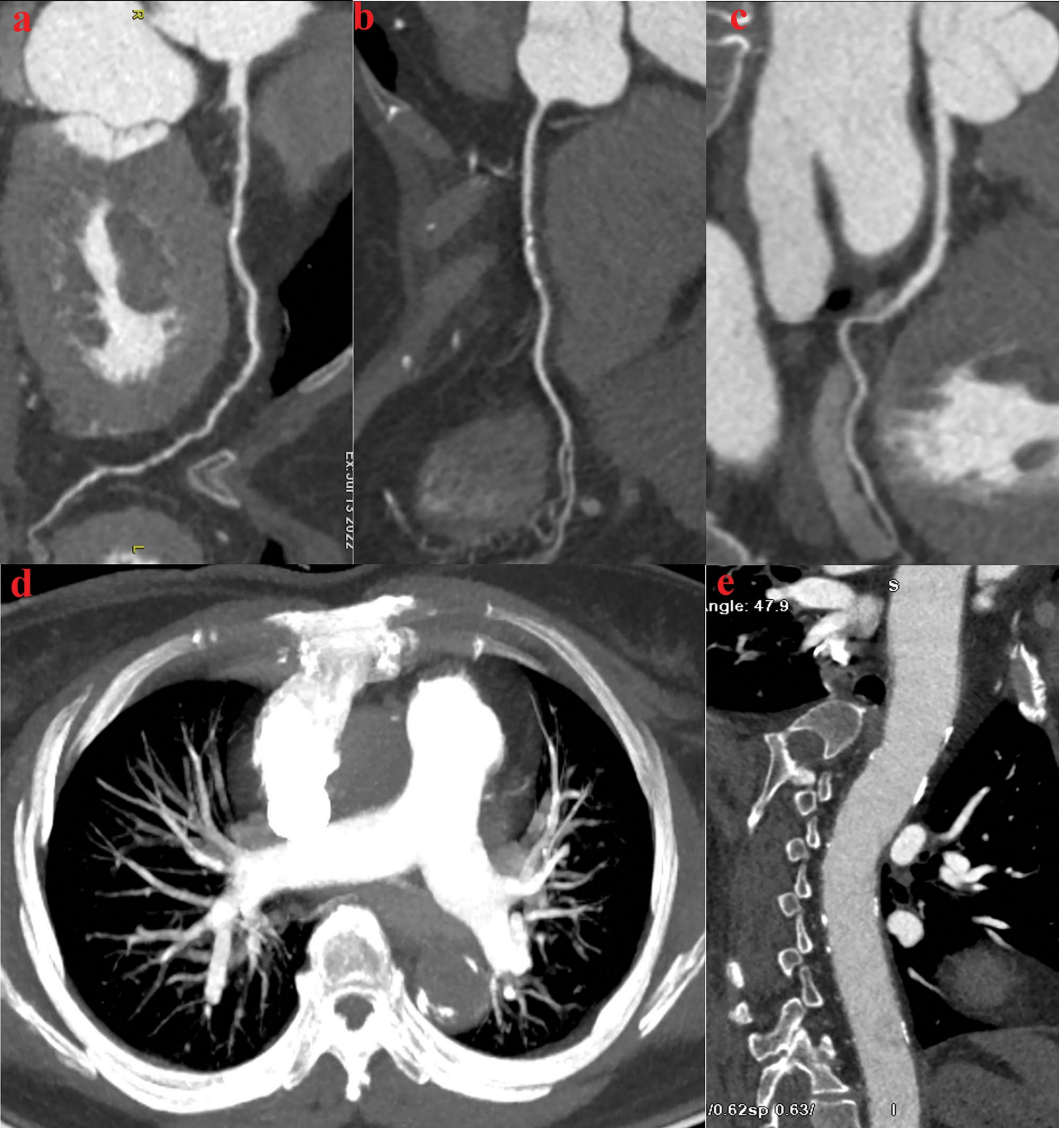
60-year-old woman with chief complaint of chest pain (heart rate: 68 bpm). Images were acquired with the optimized TRO-CTA scanning scheme. Curved multiplanar reformat CT images show the left anterior descending artery (a), right coronary artery (b), left circumflex artery (c) and the thoracic aorta (e). The maximum intensity projection (MIP) images (d) show the pulmonary artery
